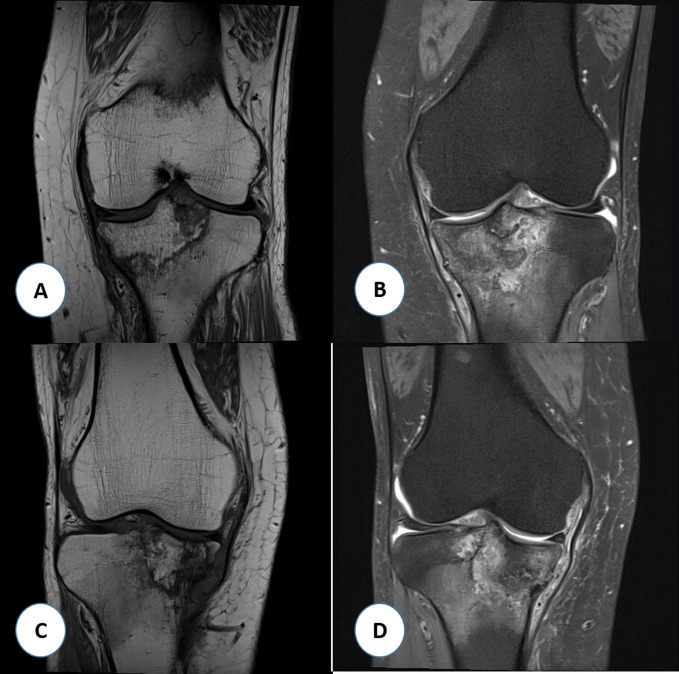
T1-weighted (A) and T2-weighted (B) MRI images of the left knee demonstrating a large area of acute bone marrow edema with abnormal signal intensity in the medial tibial plateau and irregularly contoured outline between the viable and necrotic bones. T1-weighted (C) and T2-weighted (D) MRI images of the right knee showing similar findings but more extensive to the left knee with partial collapse of the medial tibial plateau.
